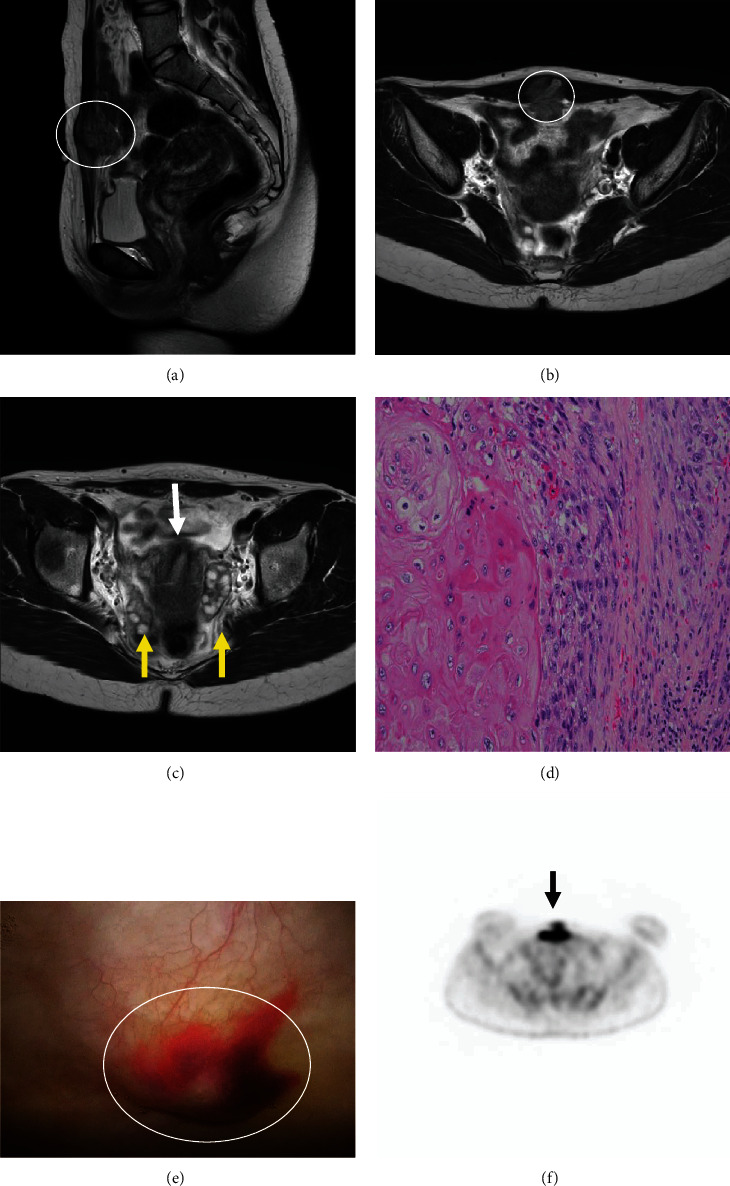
Magnetic resonance imaging showing a subcutaneous mass with an irregular surface (a, sagittal view, white circle; b, horizontal view, white circle) and with a normal uterus (c, white arrow) and ovaries (c, yellow arrows). Biopsy results reveal an invasive squamous cell carcinoma with carcinomatous nodule formation (d). Cystoscopy reveals cancerous invasion of the bladder (e, white circle). Fluorodeoxyglucose accumulation was observed in the lower abdominal wall (f, black arrows).
